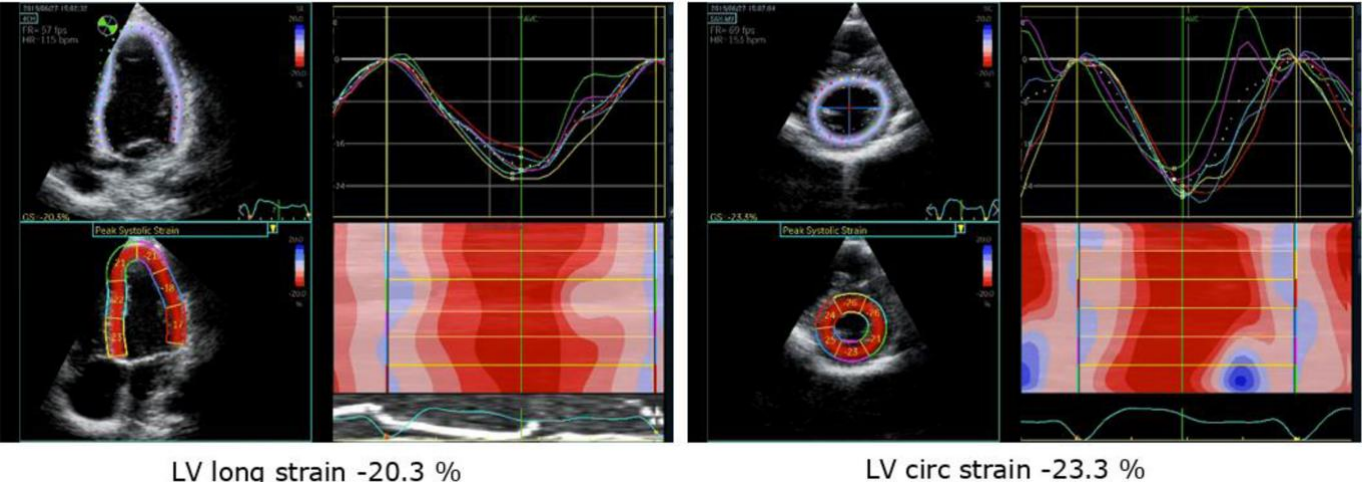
Stress echocardiogram performed at 100 W 4 months after pulmonary valve replacement in footballer.
